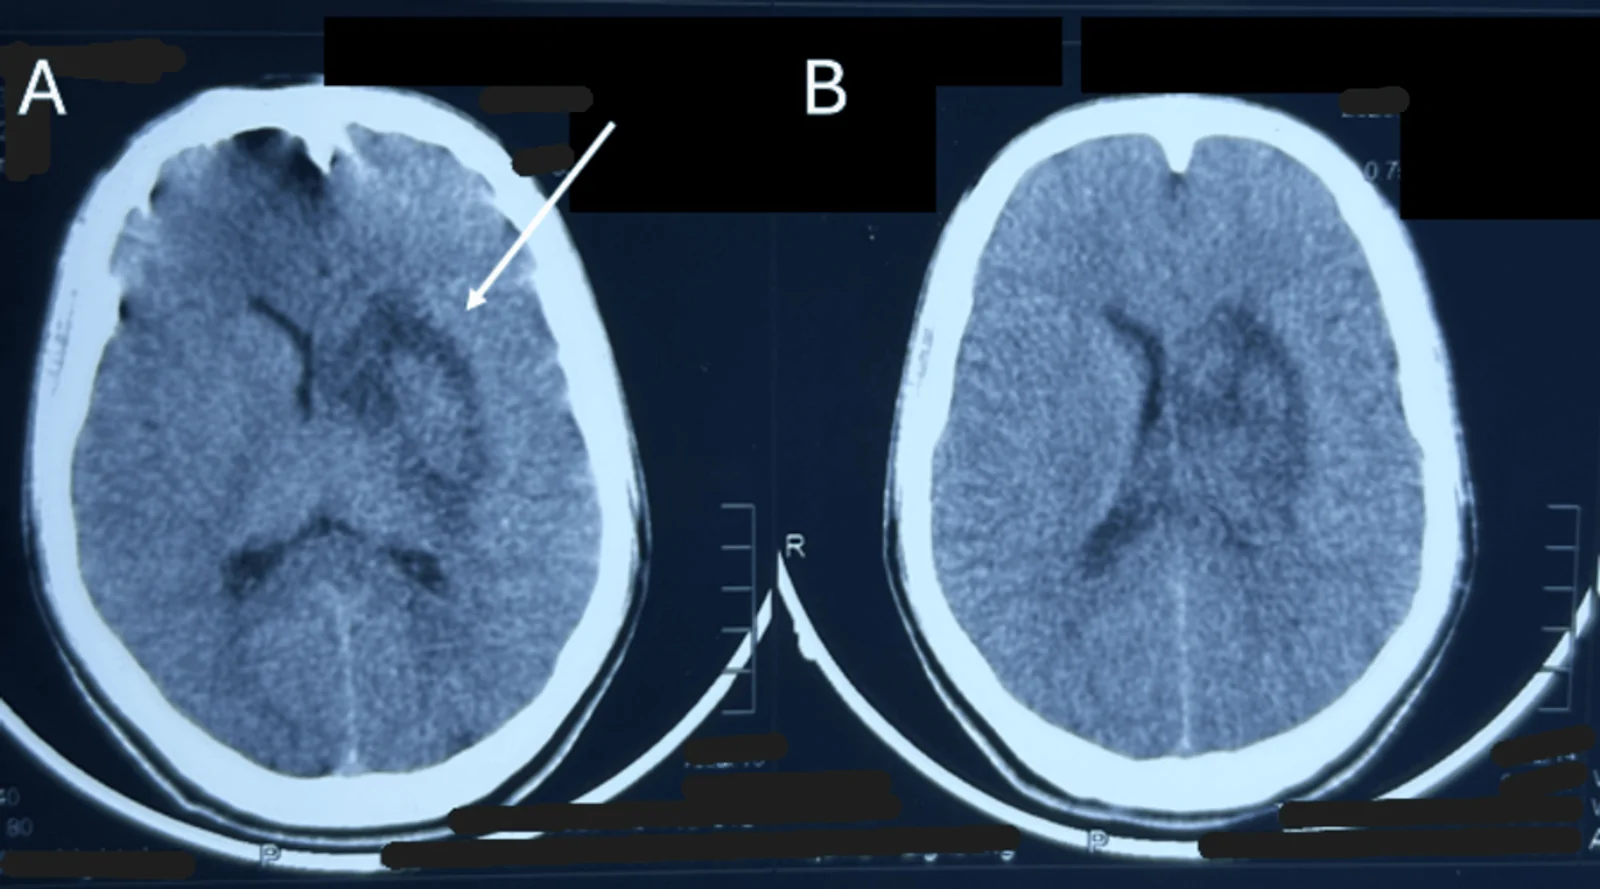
CT scan of the head showed hypodensity in the left temporoparietal region with HU 60, indicating early hemorrhagic transformation, likely an acute infarct. HU, Hounsfield Unit.
